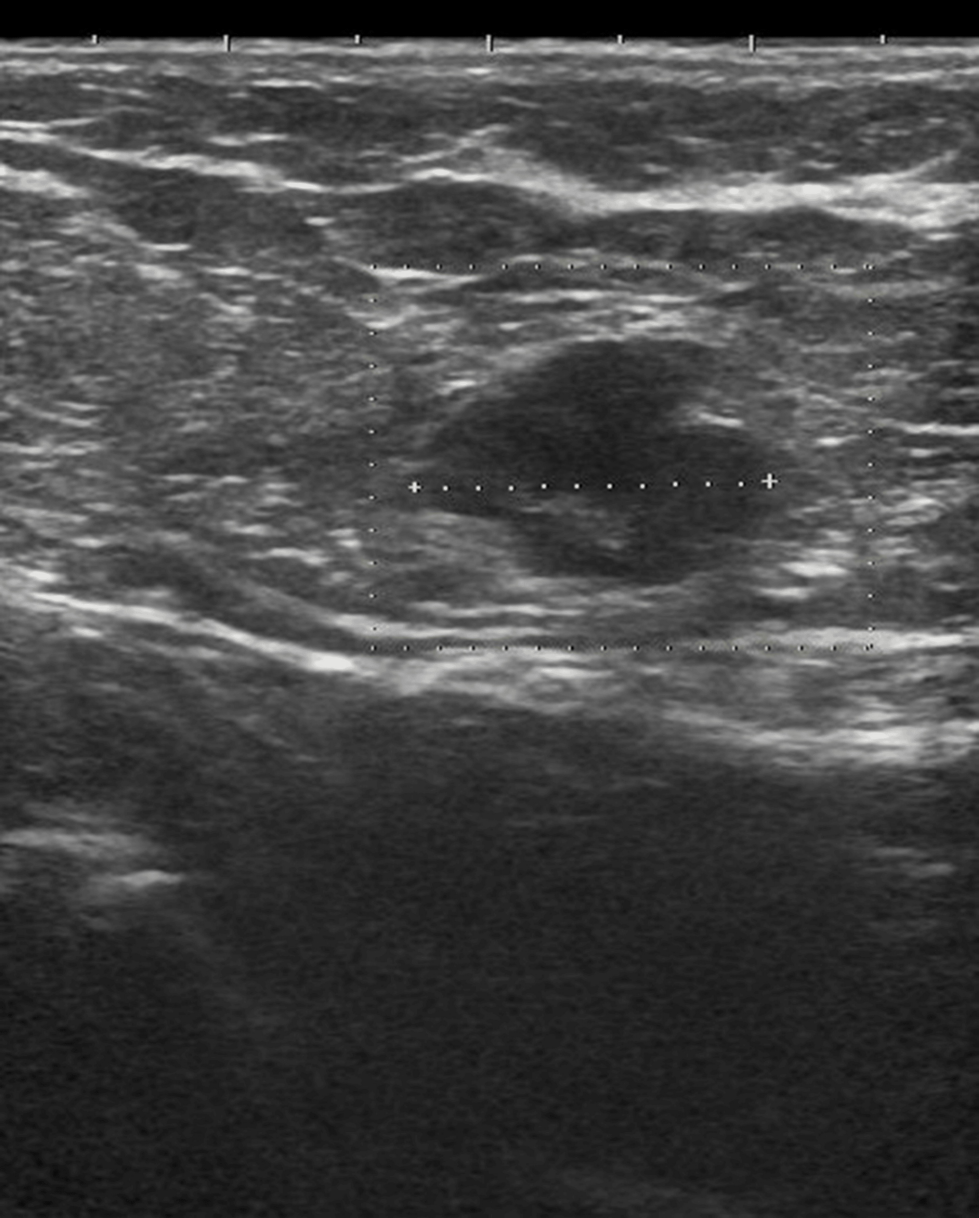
Ultrasonography Ultrasonography indicated a 14-mm lymph node enlargement suspicious for metastasis.
